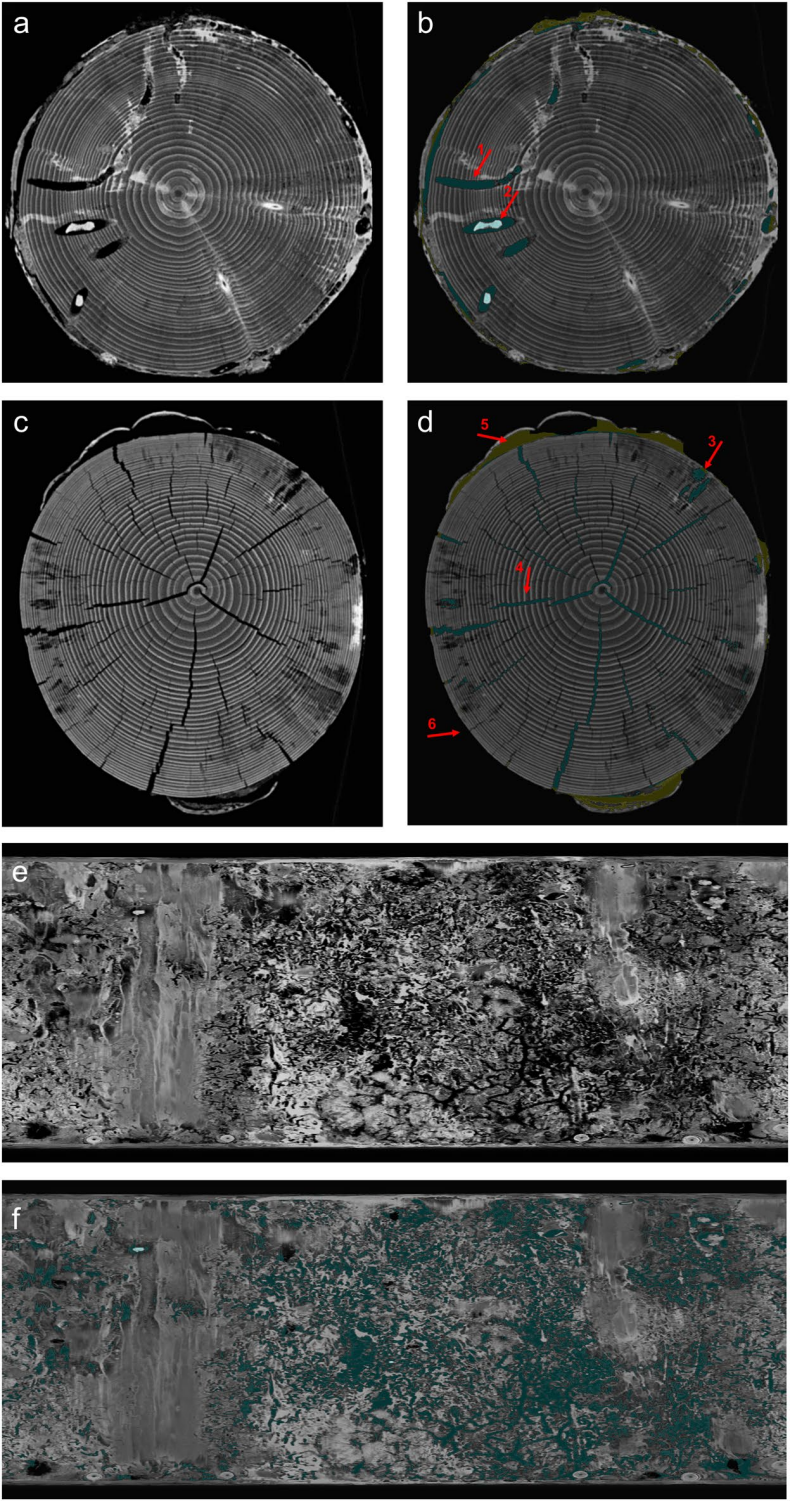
Computed tomography images of cross sections (a–d) and unrolled bark (e–f) after 1.5 years of decomposition. (a,c,e) Show raw images before segmentation and (b,d,f) show detected beetle tunnels in green and yellow. Correct identification is highlighted in (b) for beetle tunnels (1) and beetle larvae (2). Detection errors are highlighted in d including areas affected by fungal decay (3) and shrinkage cracks (4) falsely classified as beetle tunnels as well as challenges related to bark decay. Detached bark (5) can lead to overestimation of beetle tunnel volume under the bark while bark loss (6) leads to underestimation. (e,f) Show projections of the unrolled bark layer with the vertical axes representing the longitudinal stem axis.
